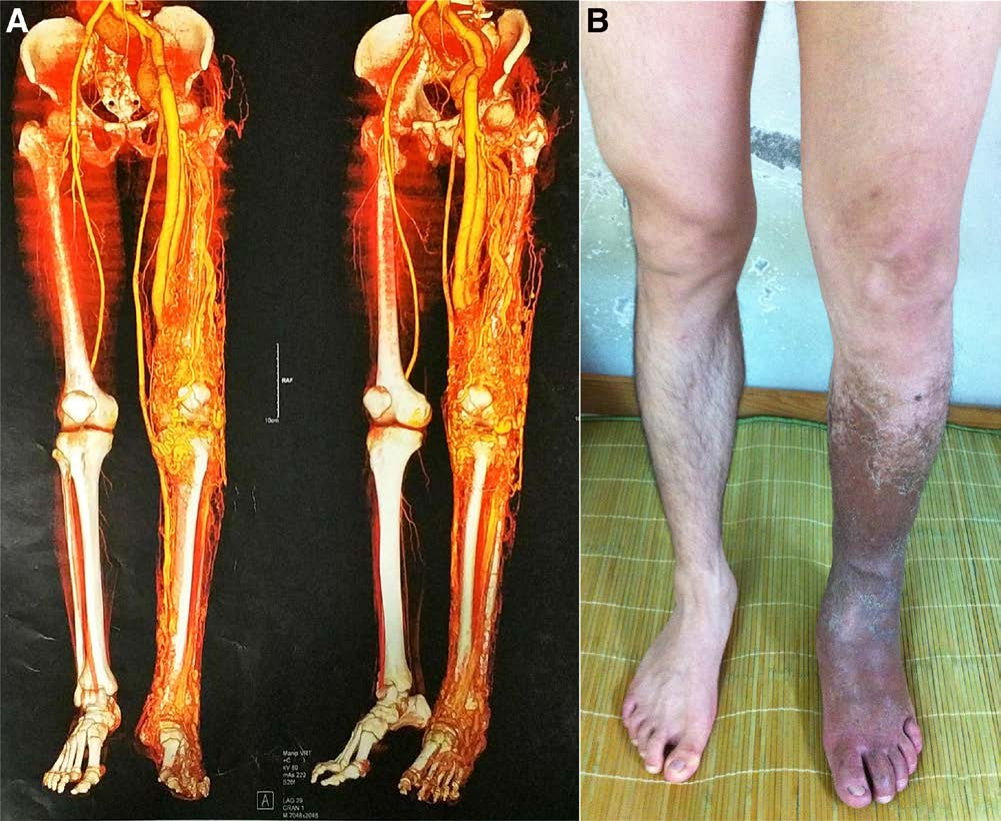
A 33-yr-old male with multisegmental spinal arteriovenous malformation and PWS. (A) The computed tomography angiography of both lower extremities of the patient. (B) Localized tissue overgrowth and localized skin pigmentation in patients. PWS = Parkes–Weber syndrome.
